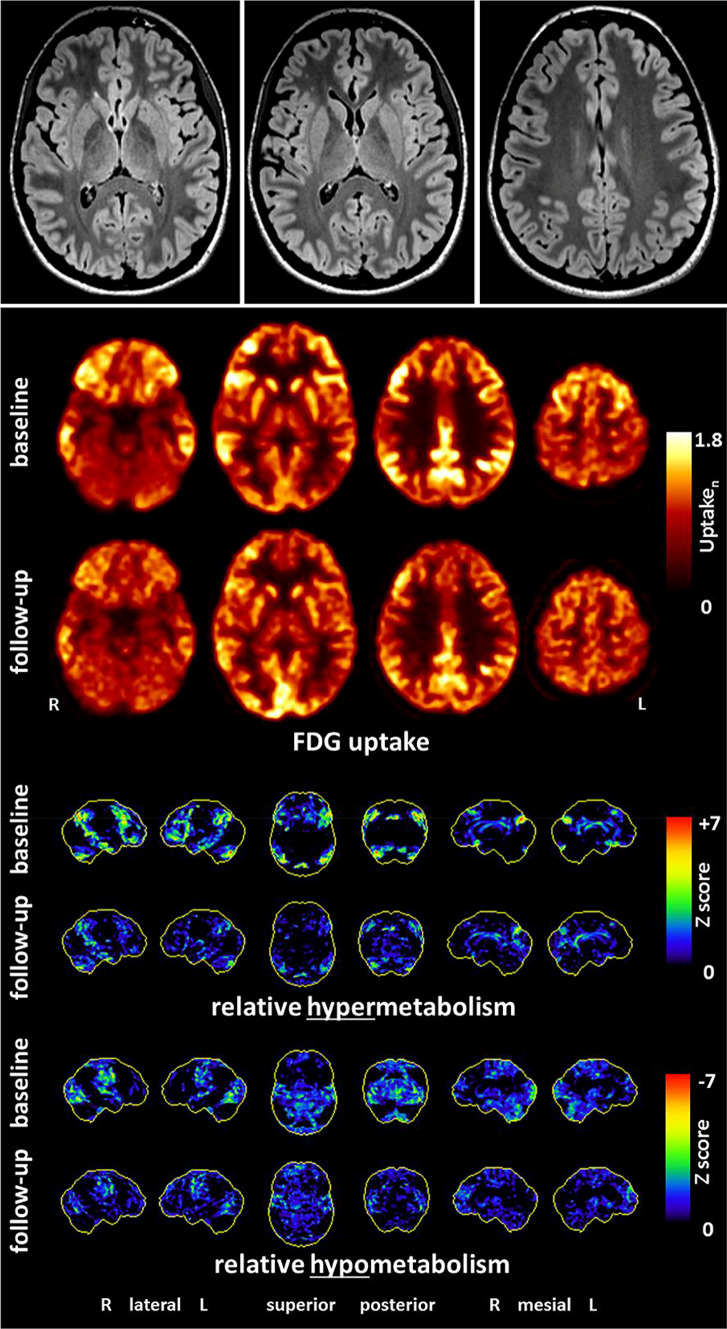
Findings of a 21-year-old female patient with probable anti-NMDA-R encephalitis. Magnetic resonance imaging depicted only a few slight, nonspecific bifrontal white matter lesions. [^18^F]fluorodeoxyglucose positron emission tomography showed pronounced relative hypermetabolism of her association cortices and a relative hypometabolism of the primary cortices (at baseline), which quickly improved during the follow-up examination after anti-inflammatory treatment (^©^Endres et al., 2019, Front Neurol. Nov 5 [28]: https://www.frontiersin.org/articles/10.3389/fneur.2019.01086/full)*Tumor screening* Tumor screening is essential in the event of the detection of paraneoplastic, onconeural antineuronal Abs.
